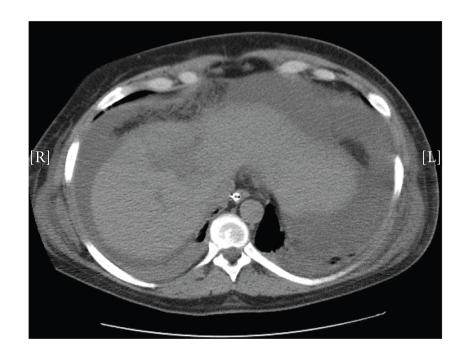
Computed tomographic (CT) scan showing a high-grade liver injury along with large amount of intraperitoneal fluid in the upper abdomen.
